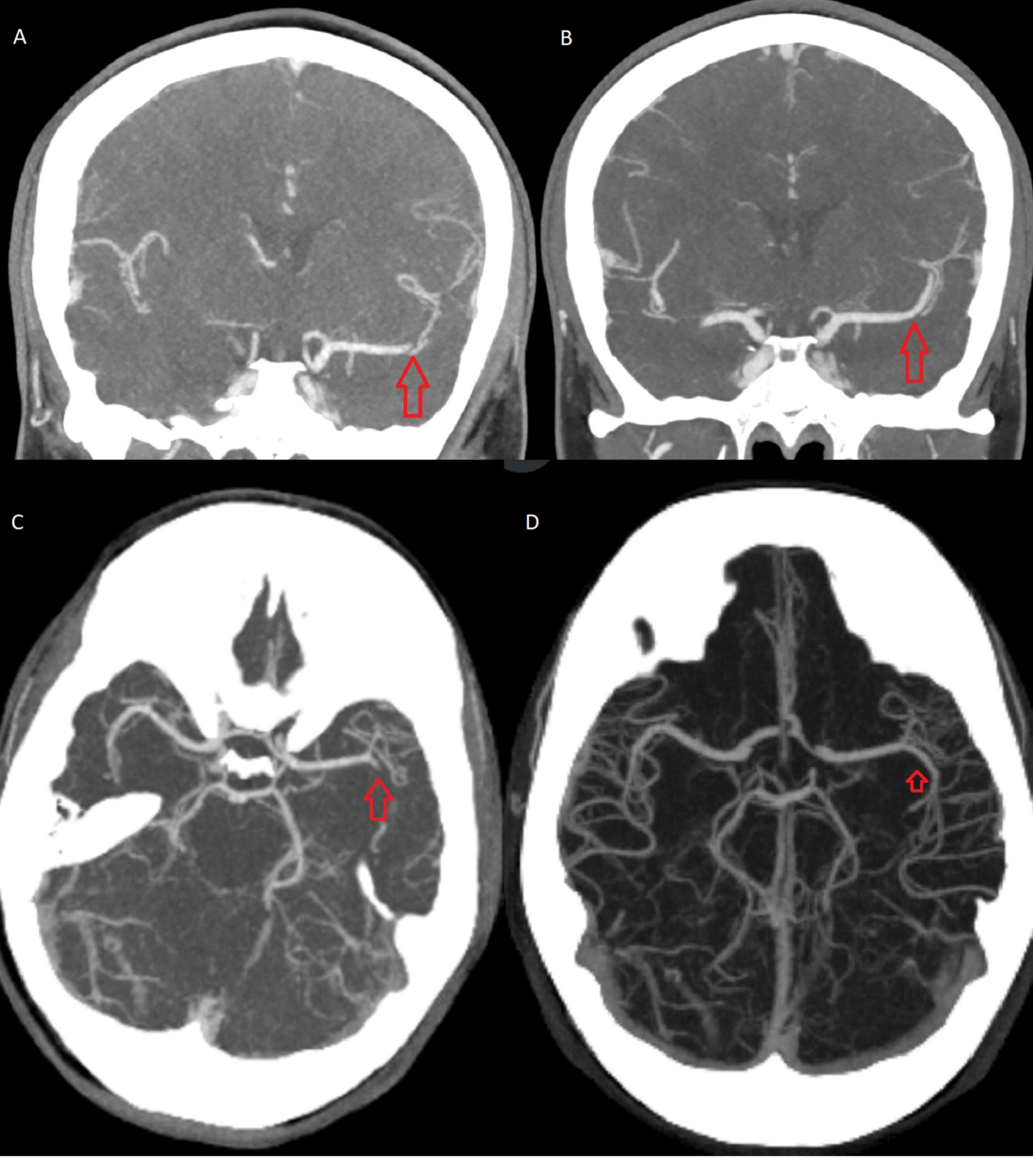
CT angiography of the brain vessels Left middle cerebral artery M2 branch thrombus (A) with maximum intensity projection (MIP) images (C) and resolution of the thrombus on follow-up imaging in three months (B) with MIP image (D).
